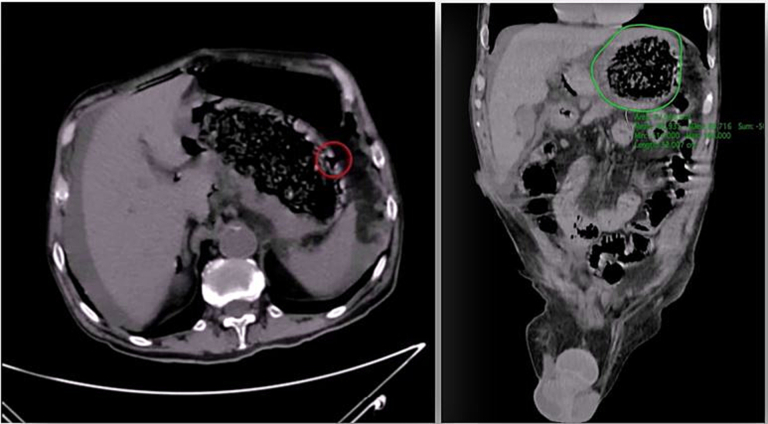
CT abdominal scan with free fluid and air in the abdominal cavity. The stomach appeared full of dense material with thickened walls.
